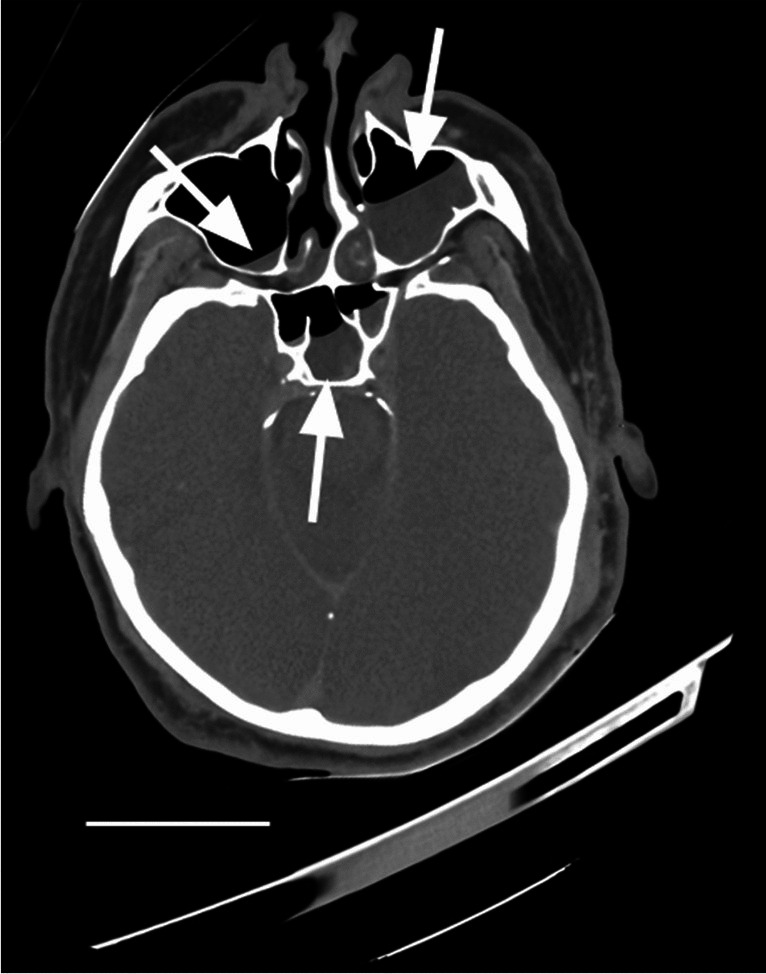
Non-drowned submerged human. Representative image of CT scan of non-drowned human submerged following homicide. White arrows indicate fluid in both maxillary sinuses and sphenoidal. Scale bar = 6 cm
